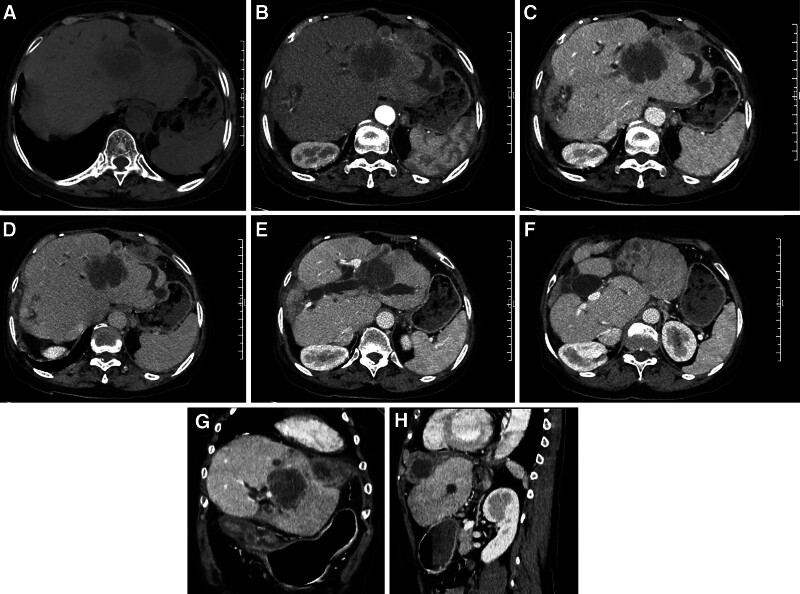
The patient’s CT scans. (A–D) Two space-occupying lesions are identified in the left lobe of the liver and subdiaphragmatic region, demonstrating low density on non-contrast CT. On contrast-enhanced imaging, the hepatic lesion displays ring-like enhancement with a central non-enhancing area and peripheral patchy mild enhancement. The subdiaphragmatic lesion exhibits thick-walled ring-like enhancement. (E) The lesion in the left lobe of the liver has encroached upon the bile duct and the left branch of the portal vein. (F) In the left lobe of the liver, there are also several small nodular ring-enhancing lesions. (G and H) The coronal and sagittal views reveal lesions in the left lobe of the liver and subdiaphragmatic region. CT = computed tomography.
